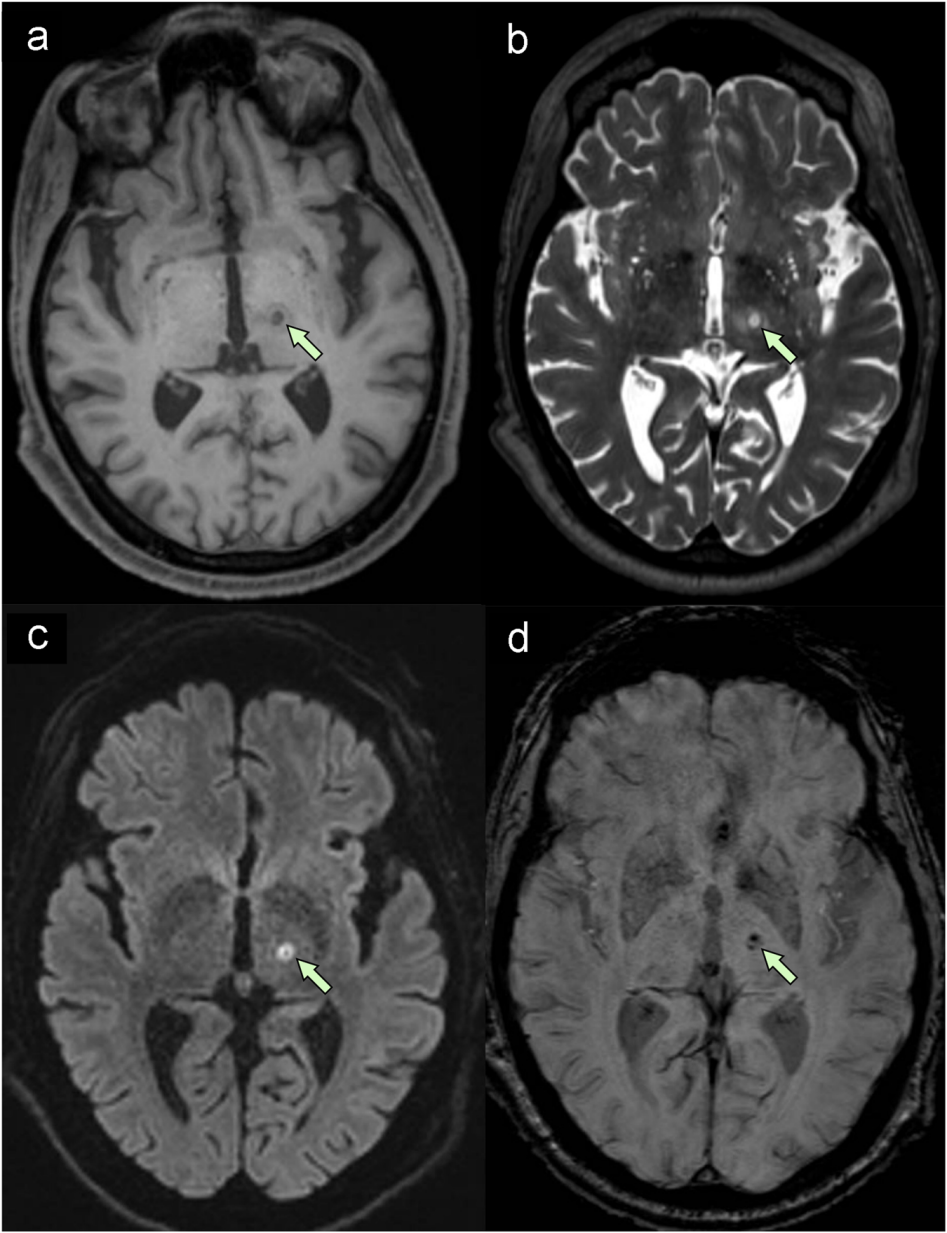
Representative MRI on postoperative day 1. a T1-weighted image shows hypointense lesion in the thalamus. b T2-weighted image shows hyperintense lesion with a small hypointense core. c Diffusion-weighted image demonstrates diffusion restriction within the lesion, suggesting tissue infarction. d Susceptibility-weighted image demonstrates hypointense blood products within the lesion.
